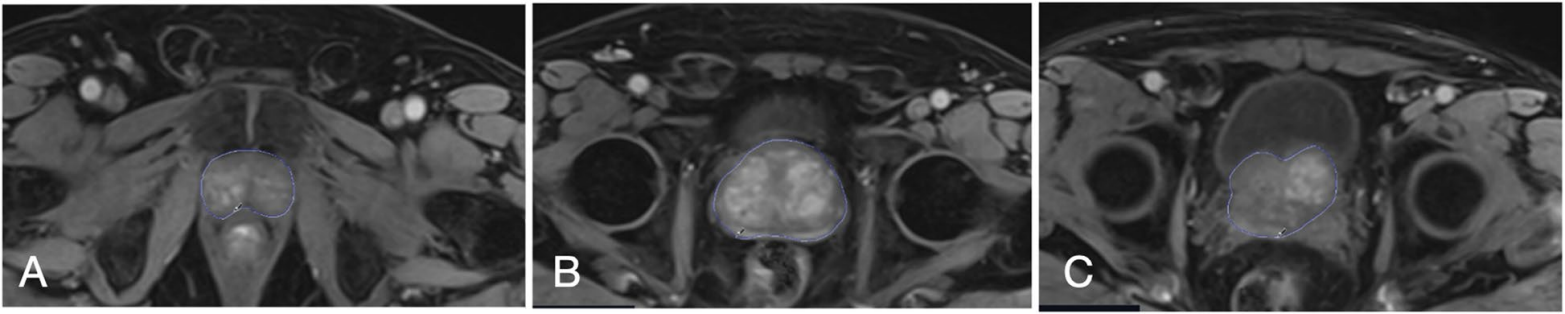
Axial T1-weighted dynamic contrast-enhanced (DCE) MRI with fat saturation following gadolinium injection, demonstrating manual delineation of the prostate at (A) the base, (B) mid-gland, and (C) apex
